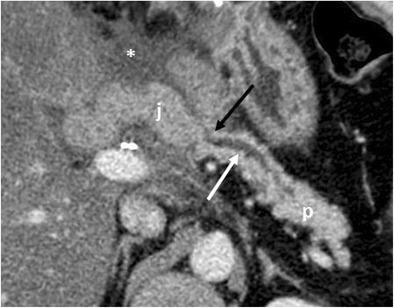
Oedema of the pancreaticojejunostomy. Axial CT image in the immediate postoperative period shows a mild thickening of the jejunum (j) at the pancreatic anastomosis (black arrow) due to acute oedema. This condition leads to a dilation of the main pancreatic duct (white arrow). A fluid collection is visible surrounding the anastomotic jejunal loop (*)
